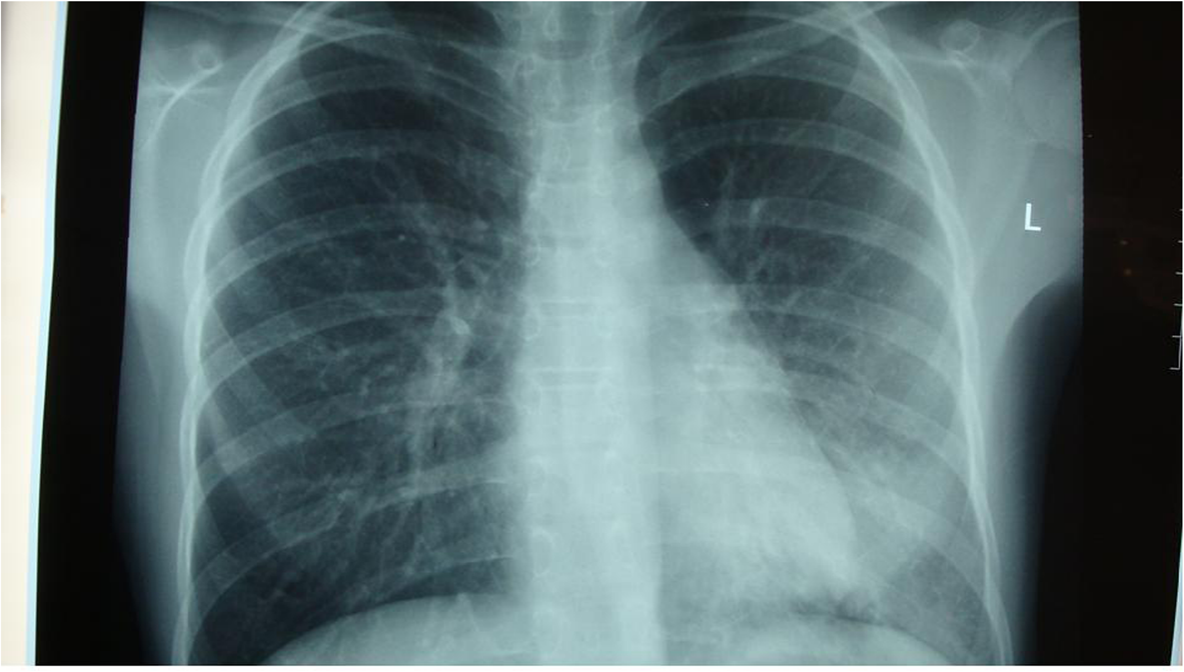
Chest X-ray revealing right lower lobe pneumonia
